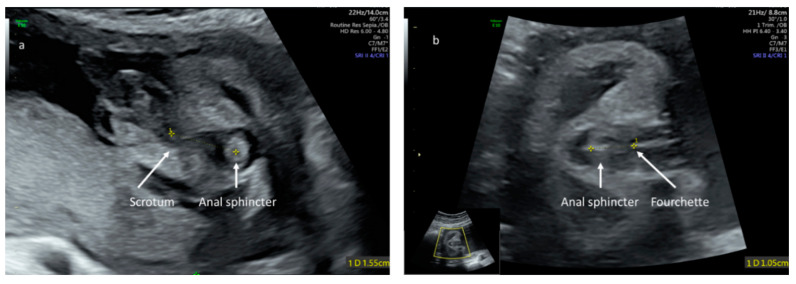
Sonographic landmarks for ano-genital distance measurement in male (a) and female (b) foetuses. In an axial view, at the level of the foetal perineum, the ano-genital distance is measured from the foetal anus to the base of the scrotum in the male or to the posterior commissure of the labia in females.
